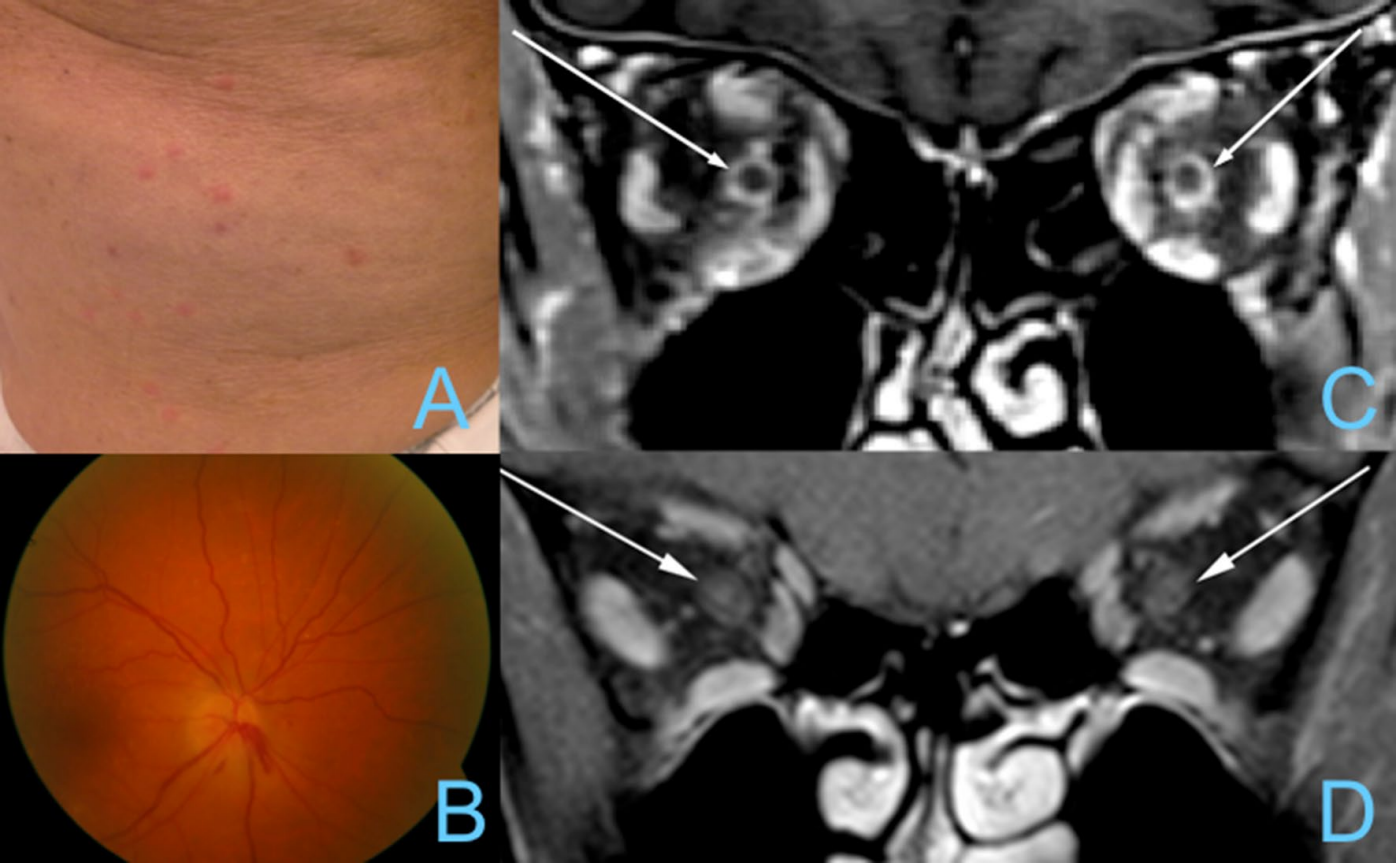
A Papular rash; B right optic nerve oedema; C patient’s optic nerve enhancement on MRI; D normal optic nerve as comparative
